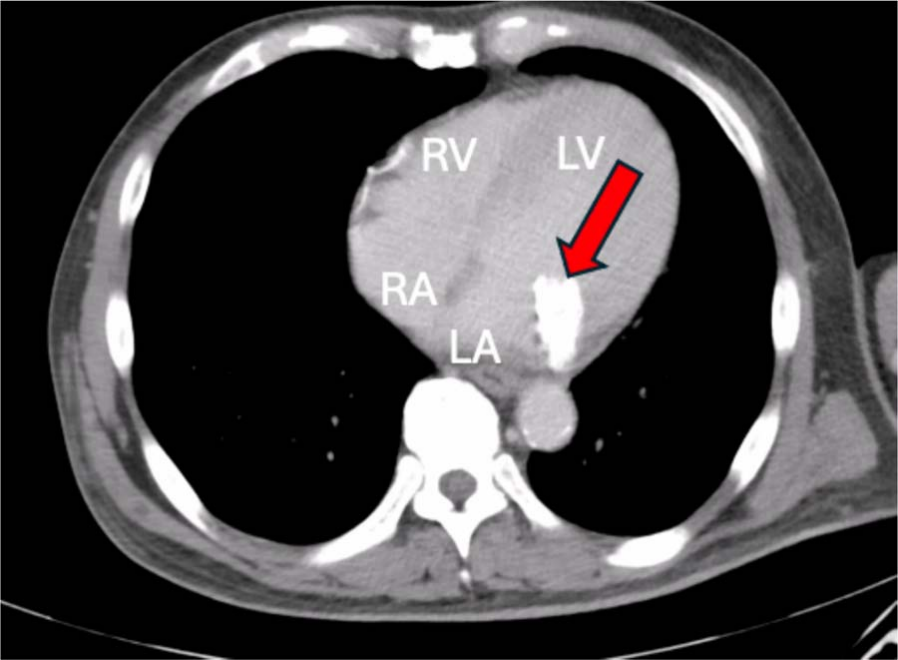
Cardiac CT showing severe MAC.
